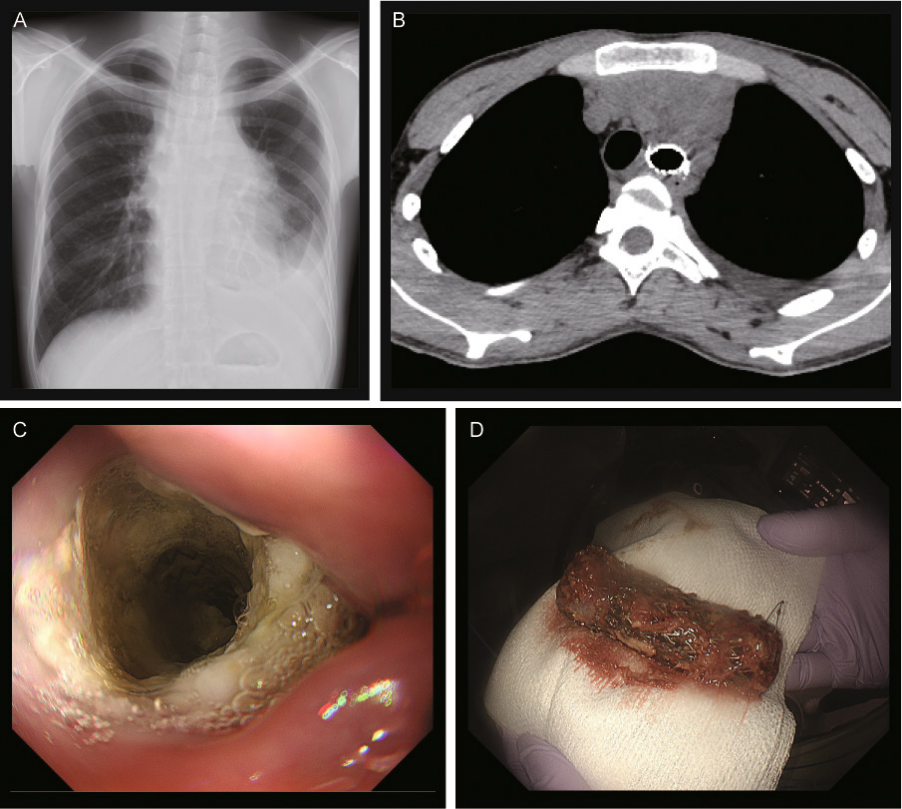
Stent ingestion findings. (A) Chest x‐ray on readmission showing the migrated stent located adjacent to the trachea. (B) Non‐contrast chest CT showing the tracheal stent within the oesophagus. (C) Upper gastrointestinal endoscopy revealing the hybrid stent lodged in the oesophagus. (D) The retrieved hybrid stent, which remained intact and undamaged after endoscopic removal.
